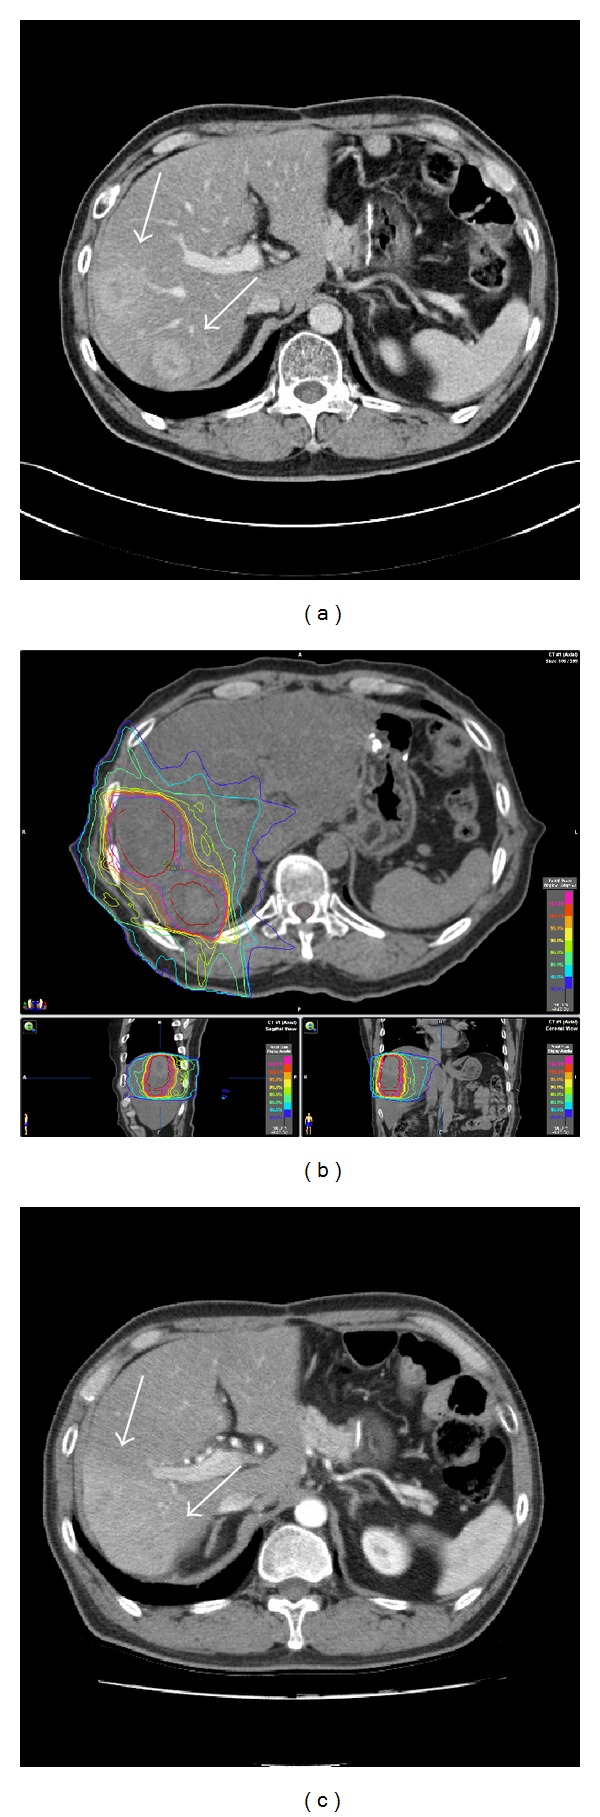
A 52-year-old male patient had been treated with surgery and postoperative adjuvant chemotherapy for sigmoid colon cancer (adenocarcinoma, T2N2M0). 13 months later, liver metastasis developed and he was then treated with salvage chemotherapy; however, follow-up CT scan after the chemotherapy showed progression of liver metastasis (white arrows) (a). We decided to treat him with SABR. The prescriptive dose to the planning target volume including two metastatic tumor lesions was 40 Gy in 4 fractions on consecutive day (b). The CT scan on 3 months after the completion of SABR showed complete response (c). Radiotherapy related change of increased density around the previous tumor lesions was shown but the patient's liver function test was normal.
